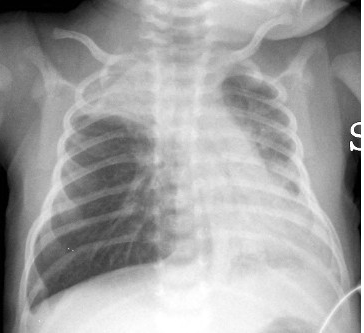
Chest X-ray on admission to the emergency room.
